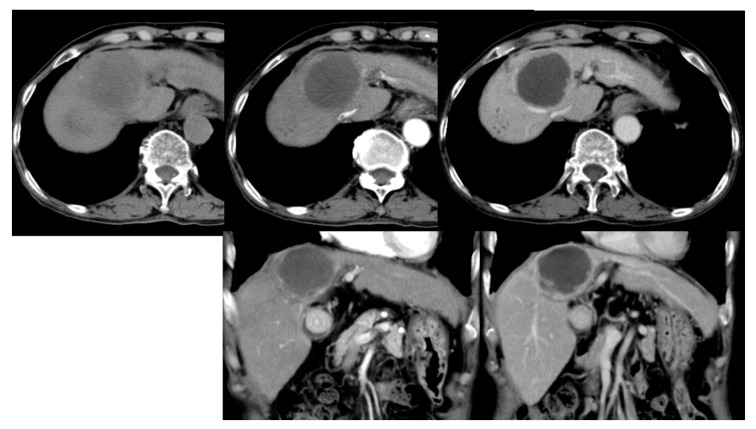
In a follow-up liver dynamic CT after treating with drug-eluting microspheres TACE, the tumor shows almost total necrosis.
